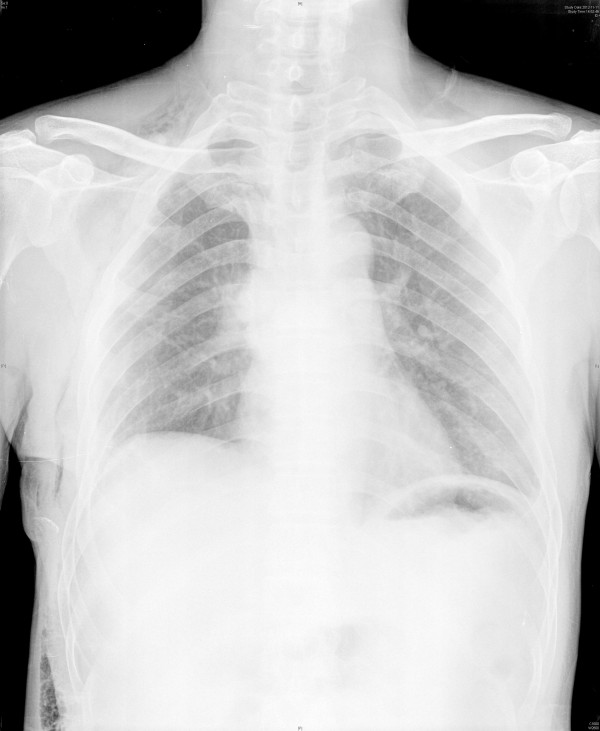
Chest X-rays showed no unnormal sign on postoperative day 7.
